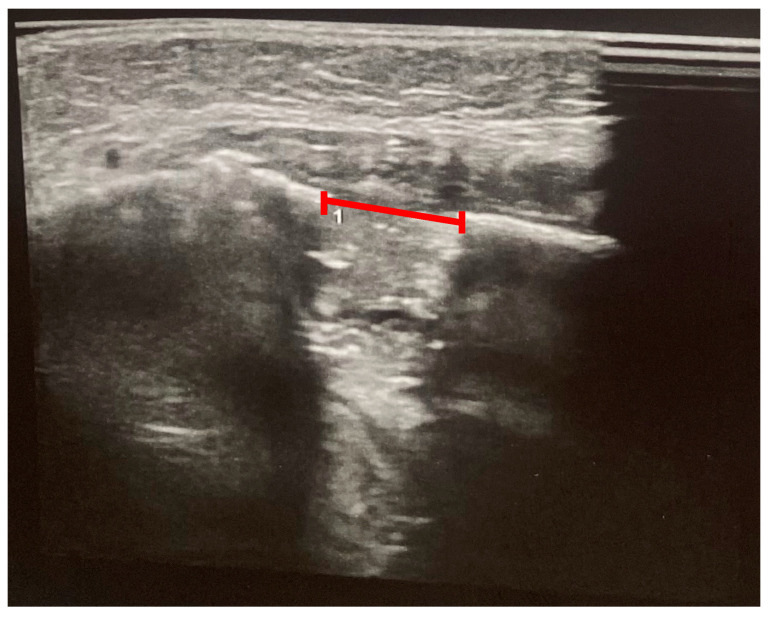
Ultrasonography at 16 weeks after completion of distraction (consolidation phase); the red line marks the residual gap between the distractors.
